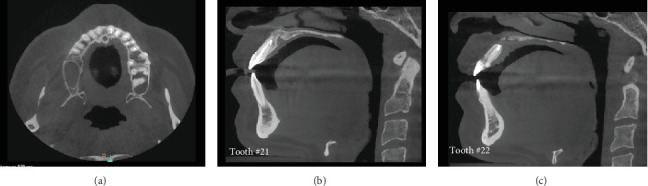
(a–c) 3-year recall CBCT radiographic evaluation of both Teeth #21 and #22; it shows partial healing around the apex of Tooth #21 and incomplete healing around the apex of Tooth #22).
